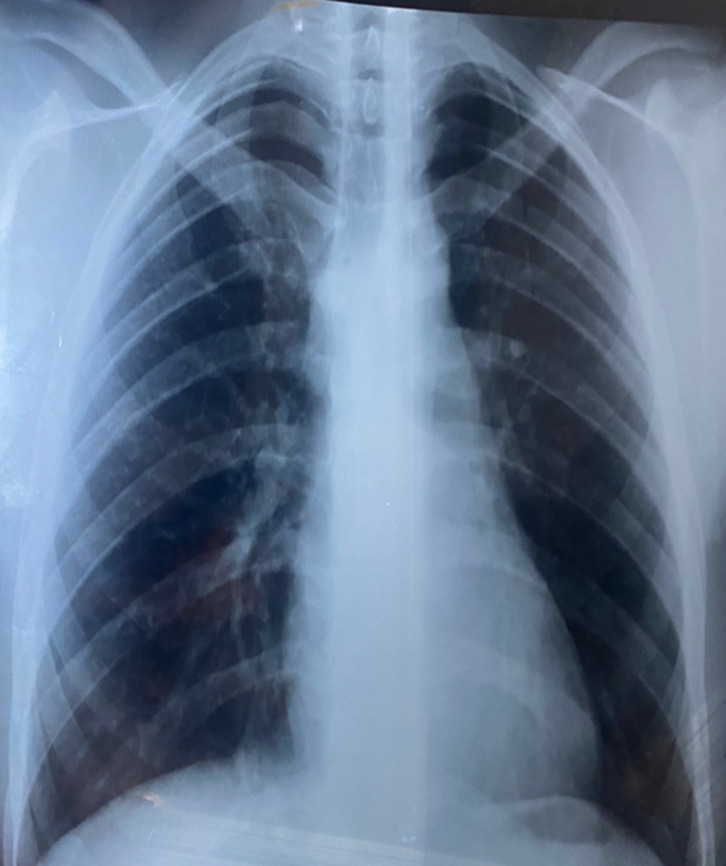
a chest X-ray showing a well-limited paracardial density of 1 cm
